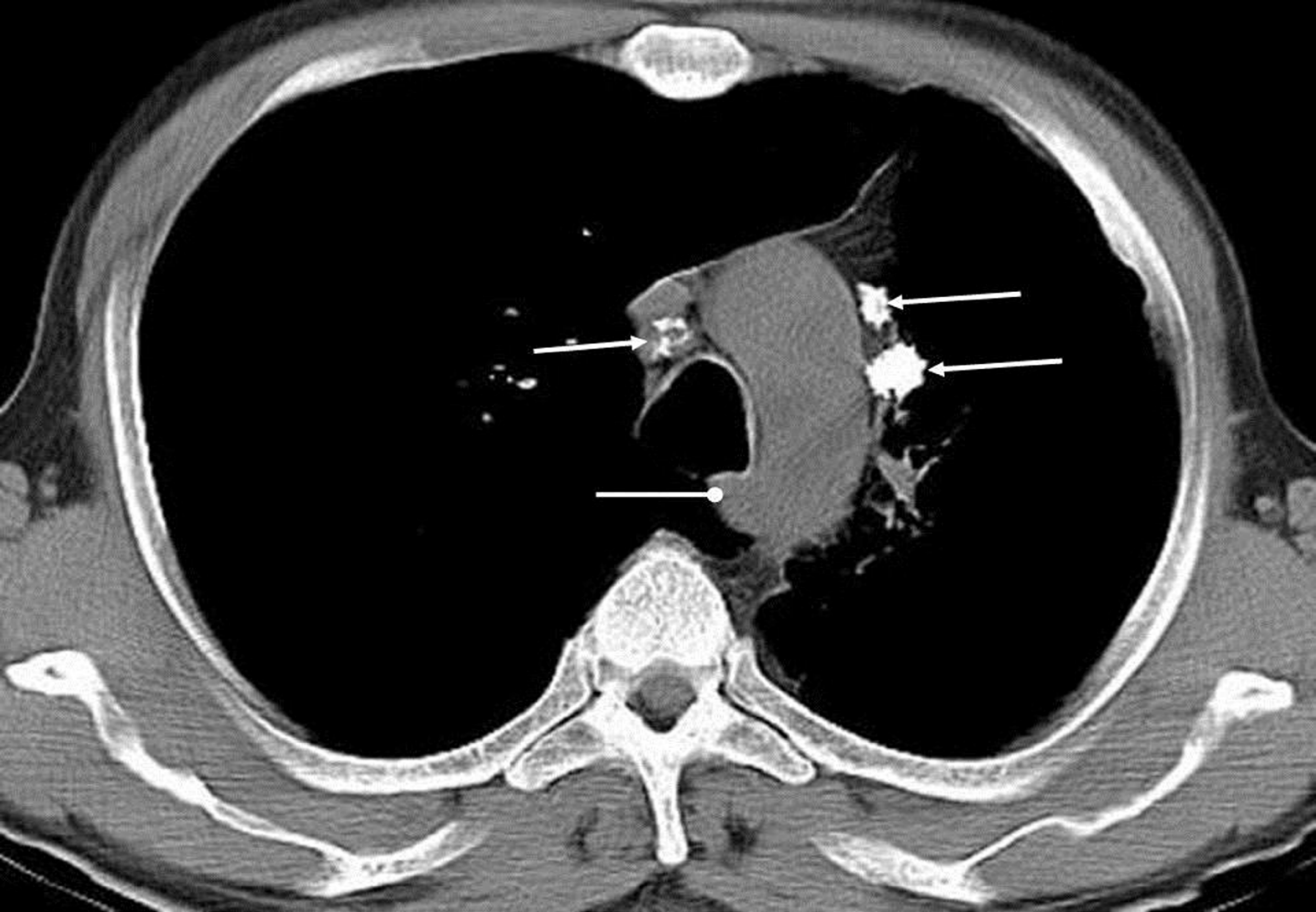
Non-contrast CT of the chest of a 65-year-old female with sarcoidosis shows multiple areas of focal calcification suggesting calcified lymph nodes (arrows) in the para-aortic and paratracheal regions of the mediastinum. The left ward deviation of the mediastinum is the result of fibrotic changes in the left lung, which can be best appreciated on the lung window. Soft tissue density seen posterior to the left side of the trachea (oval arrow) is oesophagus, which, when collapsed, can be difficult to identify.
